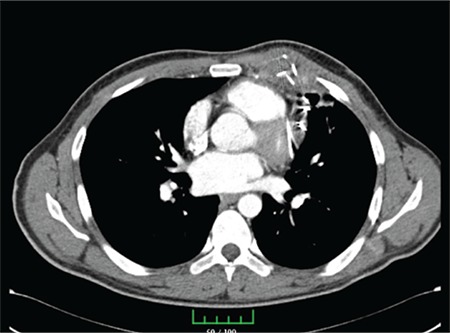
Metallic linear foreign material densities in chest wall, soft tissues and pericardial level in left parasternal area in thorax computed tomography image-mediastinum window.
